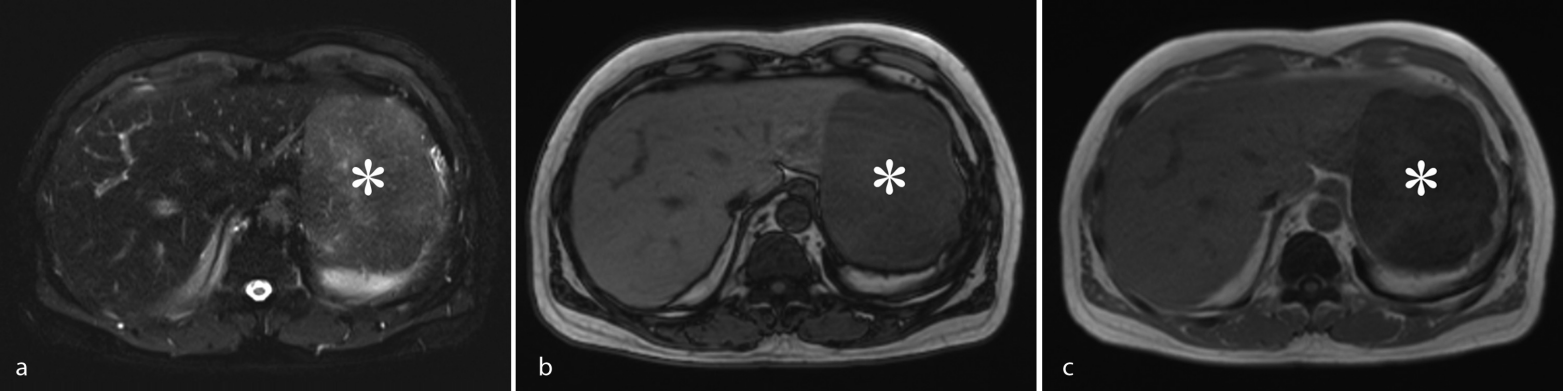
MRI of the liver shows a large mass (asterisk) at the left lobe with high signal intensity on *T*_2_ weighted image (a) and low signal intensity on opposed-phase *T*_1_ weighted image with significant signal dropout on in-phase *T*_1_ weighted image, which is representative of haemosiderin deposition (b, c).
